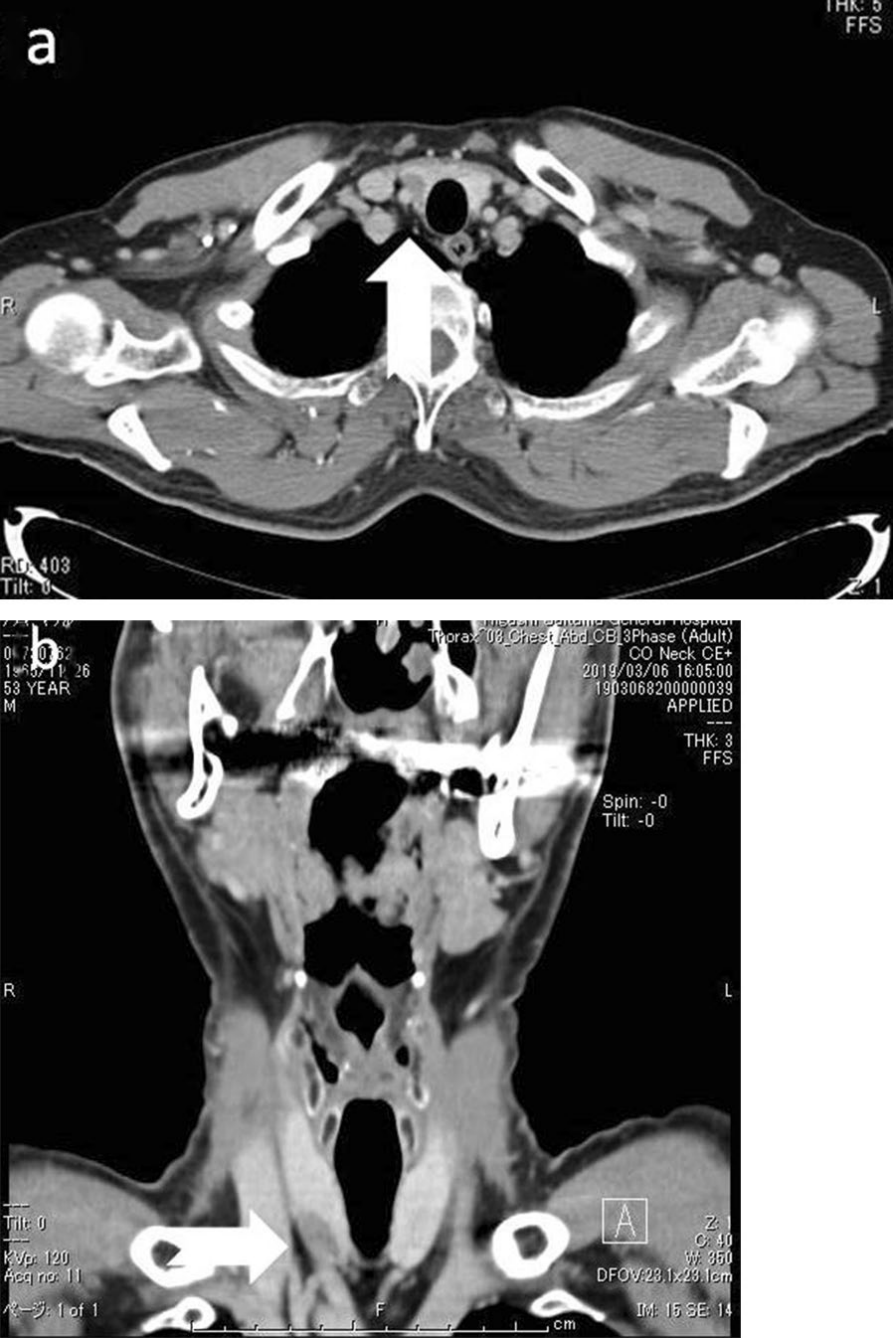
Enhanced computed tomography. a Transverse image. b Sagittal image. Images obtained by CT revealed a low density mass which was slightly enhanced by contrast injection in the right thyroid lobe
